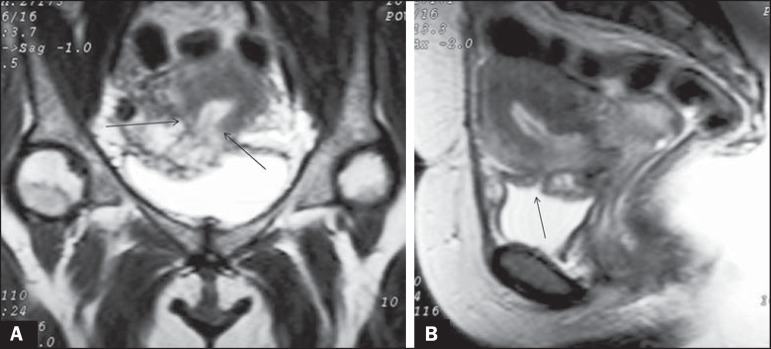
A: Coronal T2-weighted MRI showing that the myometrium
